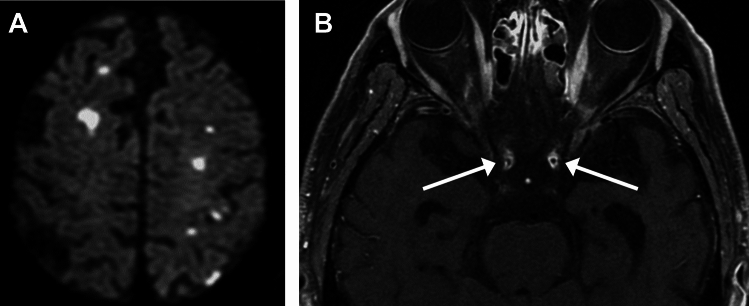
Internal carotid artery involvement and cerebral infarctions in giant cell arteritis. An 80-year-old man with GCA with impaired consciousness. (A) DWI shows multiple acute-stage cerebral infarctions bilaterally. (B) Fat-suppressed, contrast-enhanced black blood 3D-T1WI shows wall enhancement and luminal narrowing of the bilateral internal carotid arteries (arrows)
